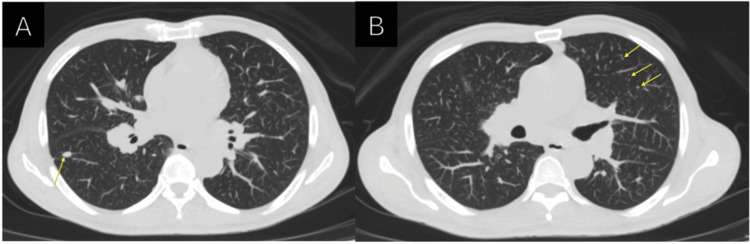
Axial view of the patient’s non-contrast computed tomography (CT) scan of the chest (A-B) Centrilobular micronodules (yellow arrows) are diffusely seen in both the upper and lower lobes of the lung.
